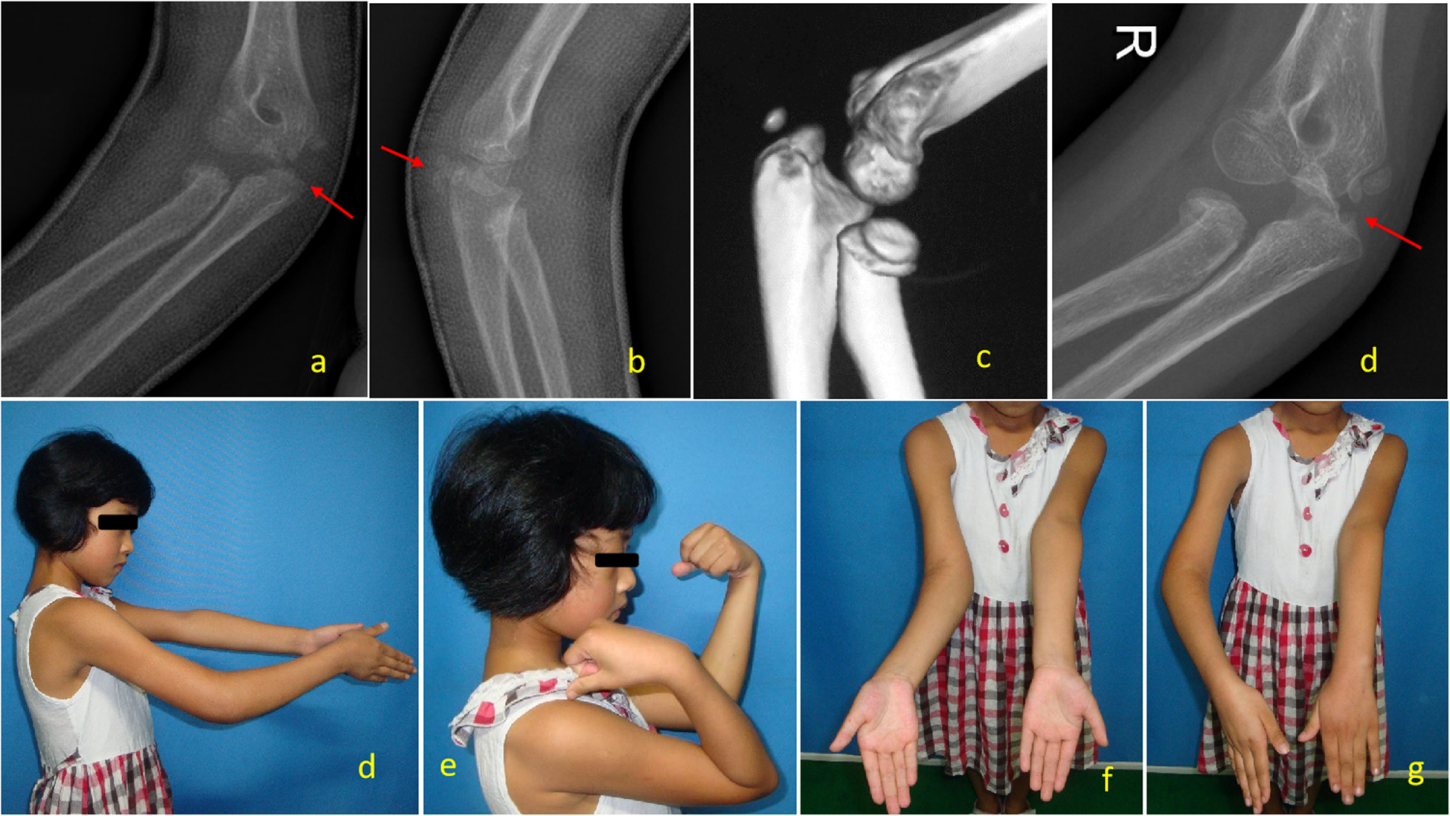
A 9-year-old girl with a type III injury in right elbow. a, b A-P and lateral view of X-ray film show olecranon avulsion fracture associated with a radial neck fracture angulated more than 30° and displacement between one third and two thirds of radial neck width. c 3D reconstructed image of CT scan demonstrate the complexity of the injury. d AP X-ray at 3 months, treated with 4 weeks of plaster immobilization only, demonstrating malunion of olecranon. e, f Twelve months after injury, the limitation of extension and g, h pronation of right elbow and forearm were observed
